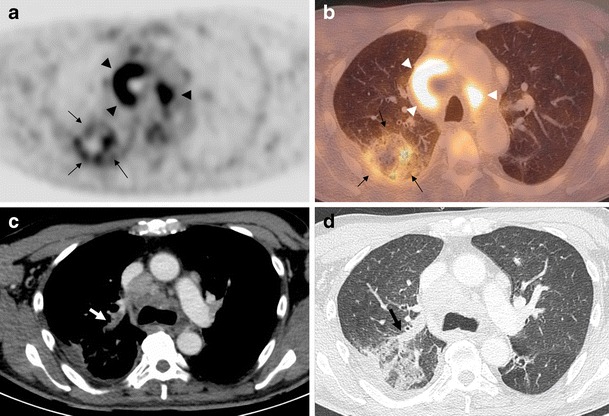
A 55-year-old man with a metastatic right lower lobe lung adenocarcinoma (patient 1). FDG-PET/CT images showed a right upper lobe subpleural consolidation exhibiting the rim sign (a, axial PET; b, axial fusion, *arrows*). Metastatic mediastinal adenopathies were clearly visible (*arrowheads*). Combined enhanced MDCT confirmed the acute pulmonary embolism (c, *arrow*) and showed the vessel sign associated with the right pulmonary infarction (d, *arrow*)
